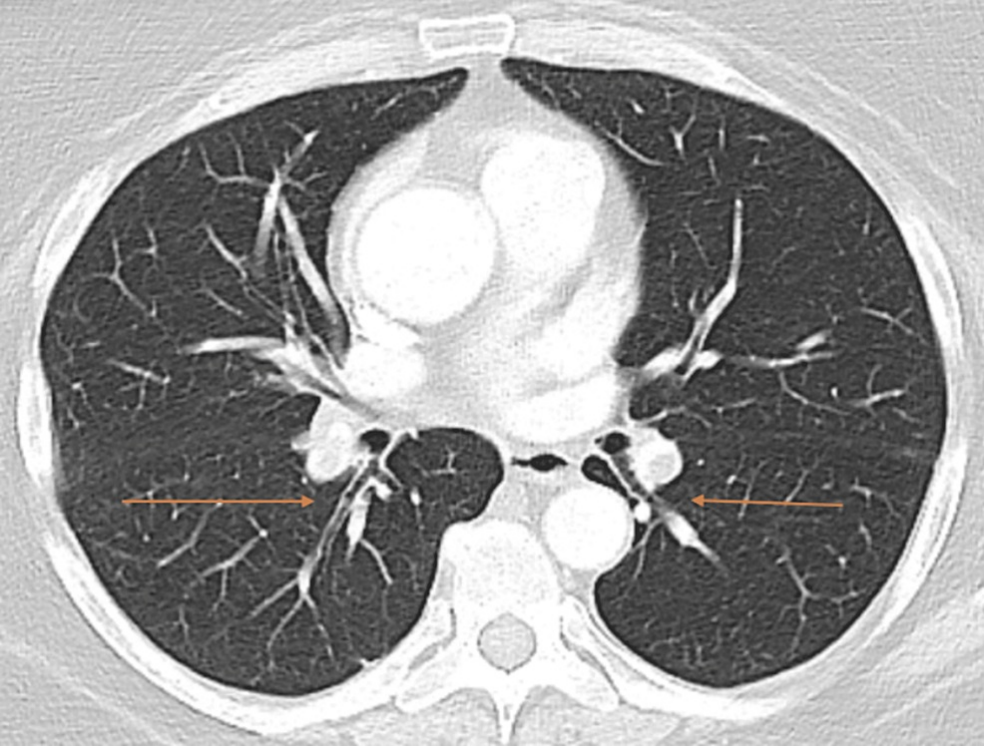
CT angiogram String-like filling defects noted in bilateral posterior pulmonary artery segmental branches.
